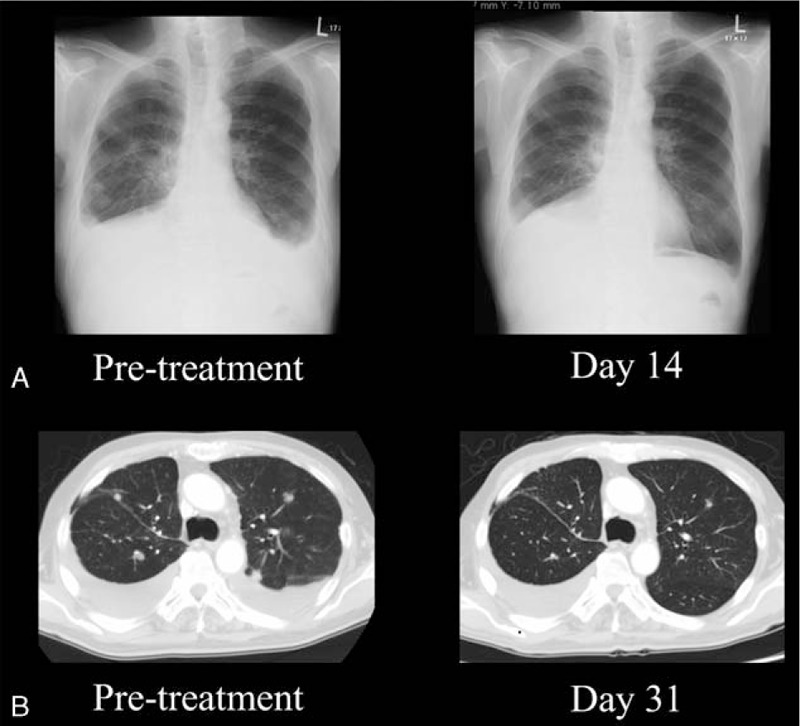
Chest x-rays and axial chest CT images of case 2. Decrease of pleural effusion and shrinkage of pulmonary nodules on chest x-rays after 14 days osimertinib administration. Shrinkage of multiple lung metastases and decrease of left-sided pleural effusion on chest CT images after 31 days osimertinib administration.
